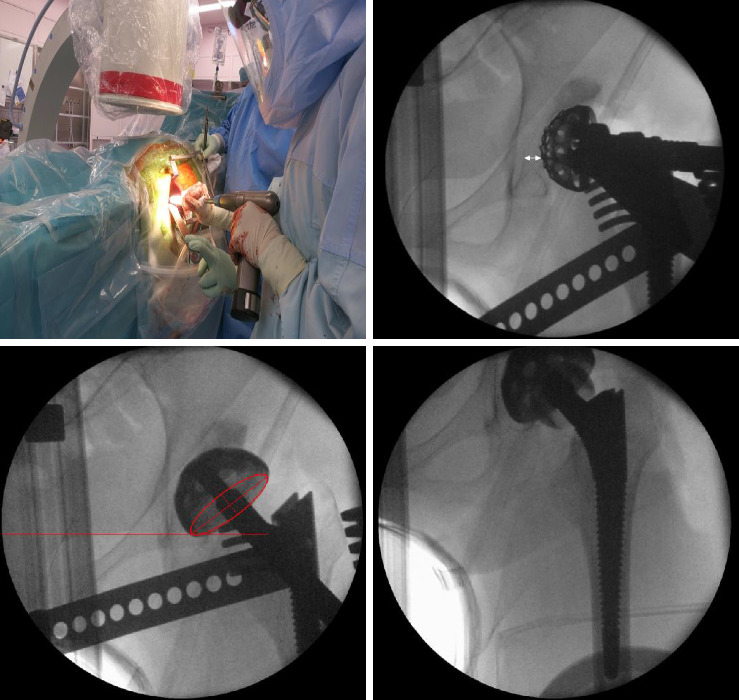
Direct anterior approach using fluoroscopic image allows assessment of reaming and placement of the acetabular cup. The depth of acetabular was evaluated before reaming (white allow). Radiographic inclination and anteversion of the cup were adjusted under fluoroscopic guide (red line). Stem alignment was checked using final broach.
